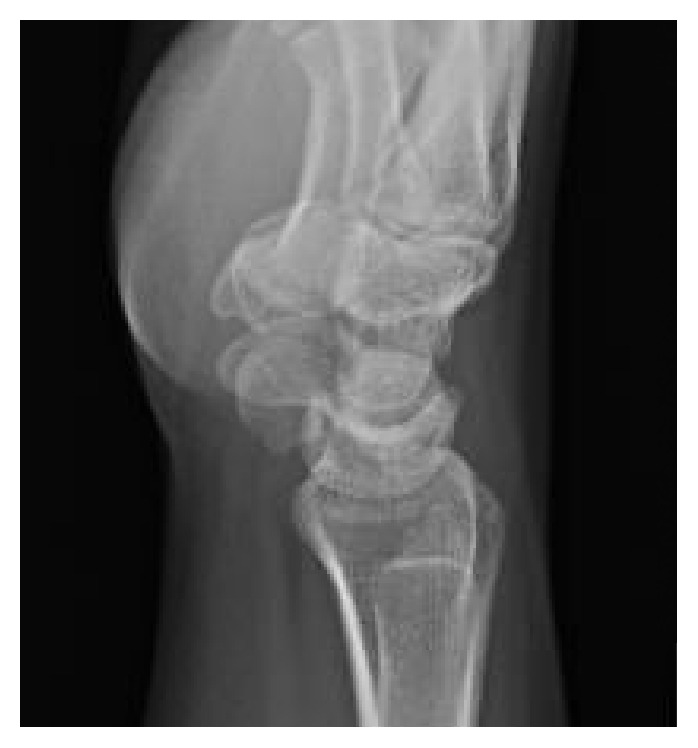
A lateral radiographic view obtained at 6 weeks after initial presentation showing a normal appearing scaphoid.
